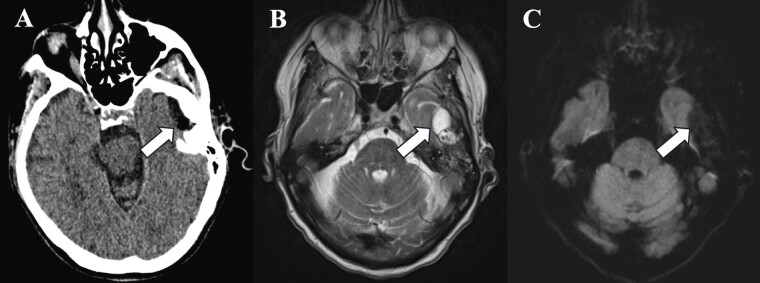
Case 1 axial postcontrast CT (
